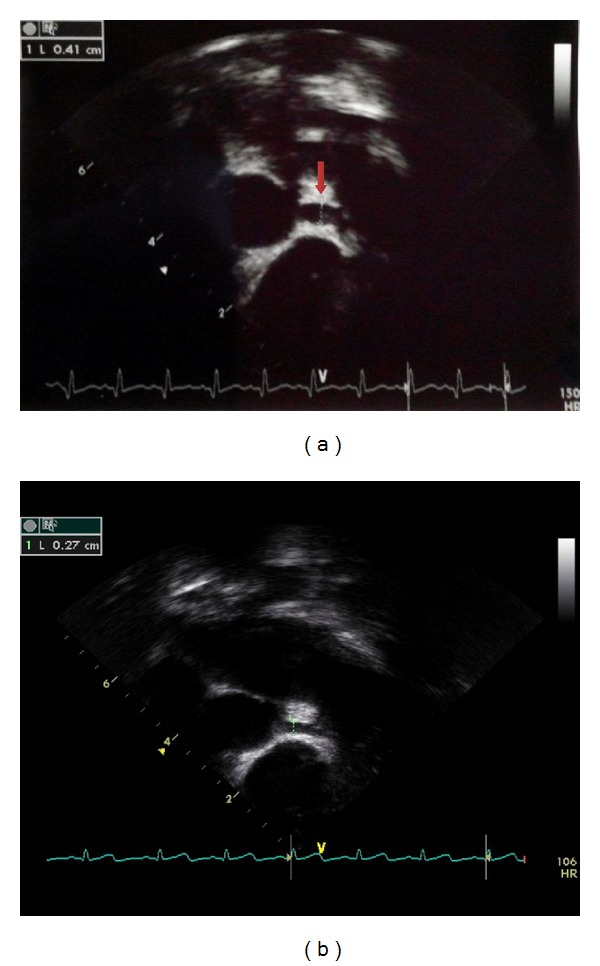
The echocardiographic findings on the 16th day of admission show fusiform aneurysm (4.1 mm) of left main coronary artery (a) but mild dilatation of left main coronary artery (2.7 mm) after the 4th IVIG  treatment (b).
